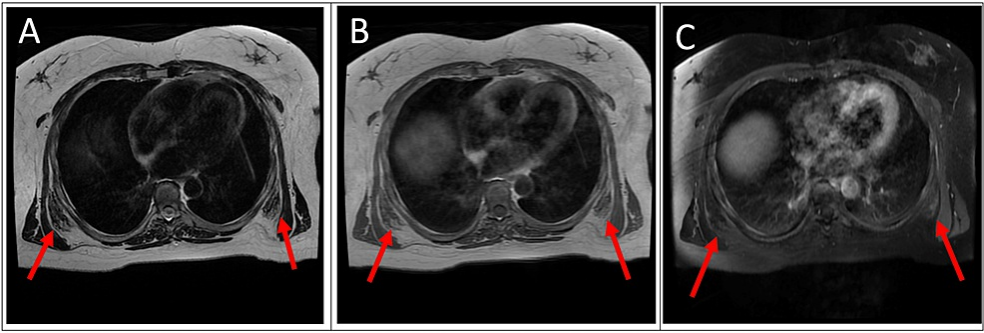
Axial (A) T2-weighted MRI reveals alternating soft tissue and fatty components of bilateral subscapular masses. Axial (B) T1-weighted MRI of bilateral suprascapular masses containing alternating soft tissue and fatty components. (C) T1-weighted post-contrast image with fat saturation showing low-level enhancement.
